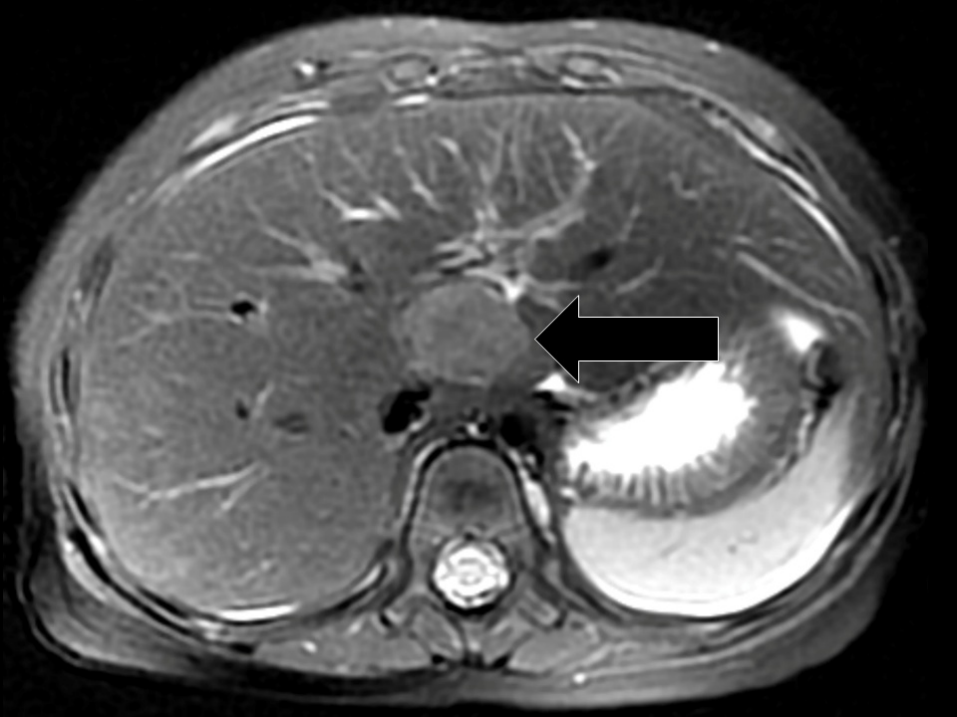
Axial T2-weighted Sequence Axial T2-weighted sequence showing a more centrally located liver lesion (indicated by arrow). While this particular lesion was the most conspicuous by MRI, it was inconspicuous by ultrasound.
